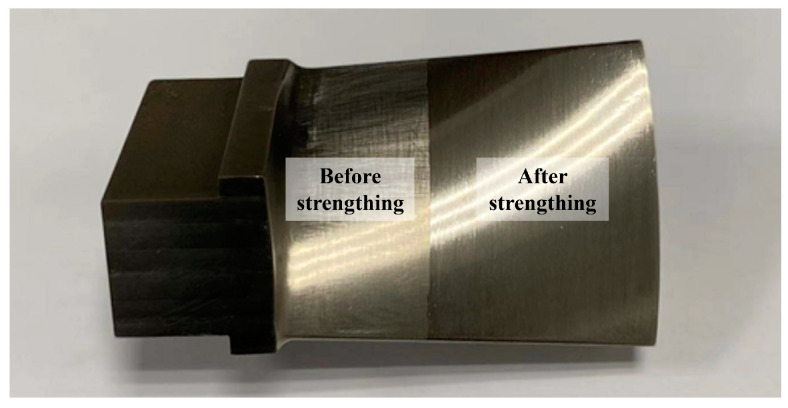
Aviation blade surface before and after ultrasonic surface strengthening.
